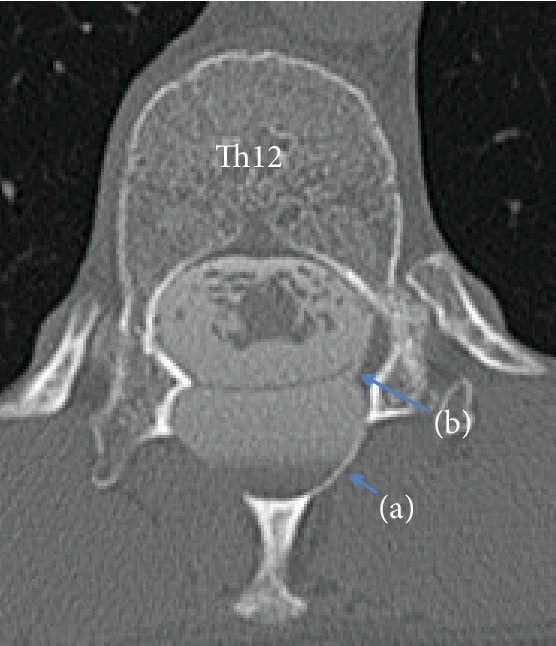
CT after myelography showed scalloping of the vertebral arch at the T12 level, with contrast medium flowing into the arch. (a) A communication pathway was present between the dural canal and the cyst at the T12 level (b).
